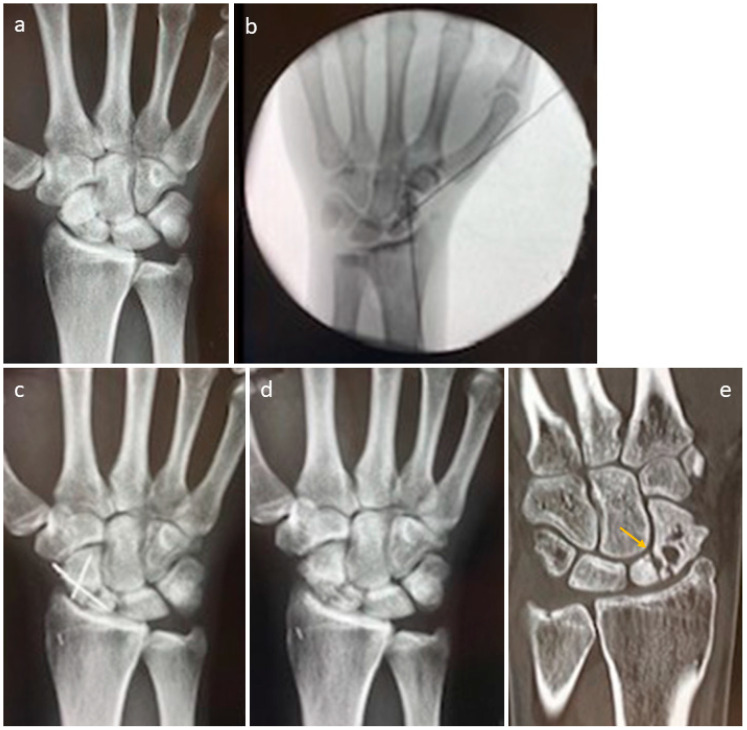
Non-union of the scaphoid after conventional treatment, even though autograft was used. (a): Pre-revision X-ray. (b): Intraoperative fluoroscopy using a vascularized femur condylar span which was attached to the aorta radialis. The span was stabilized with four K-wires. (c): Twelve weeks after surgery with the non-union still visible. (d): Shock-wave therapy did not improve the situation after eight months. (e): Five years after revision; the necrosis (orange arrow) of the proximal pole is visible, but the patient is nearly pain-free.
